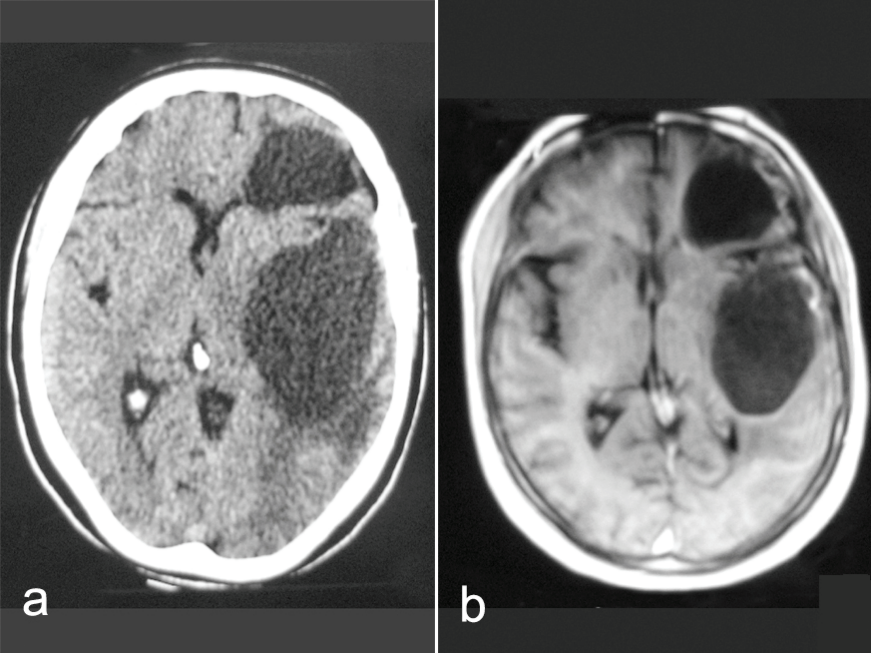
(a) Cranial CT scan showed hypodense lesions in the left frontal lobe and in the left temporal lobe. No significant edema was observed around the lesions, which compressed the left lateral ventricle and did not show contrast enhancement. (b) Cranial MR imaging showed in the left frontal region and in the left temporal region cystic lesions.
